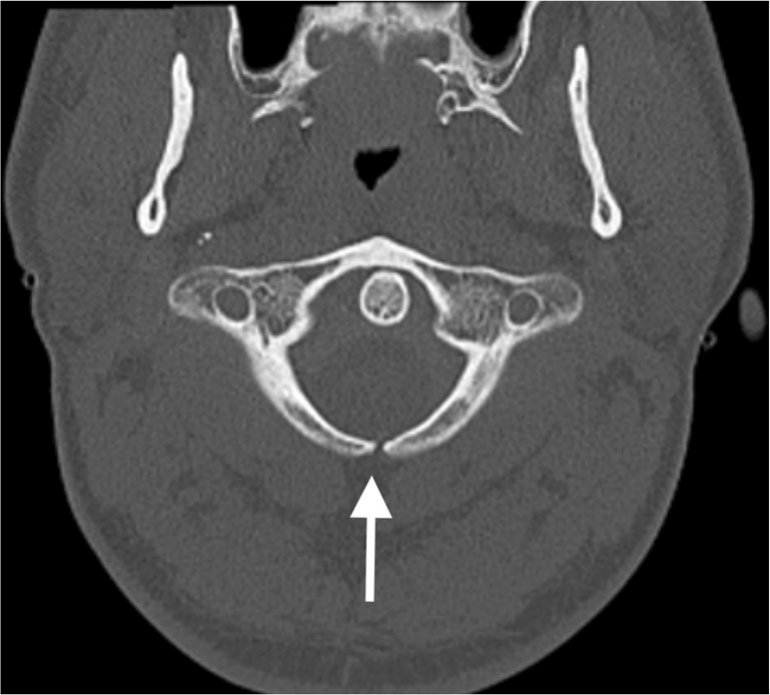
Posterior rachischisis: axial CT image through the atlas (C1 vertebra) of the craniocervical junction of a road traffic accident victim (pedestrian versus car with “bullseye” impact of the victim’s head against the windscreen of the car) demonstrating a developmental posterior rachischisis (*arrow*) which should not be confused with an acute fracture. The well-corticated margin of the cleft should alert one to the developmental nature of the anomaly in addition to the typical location and unity of the defect compared to a traumatic aetiology
